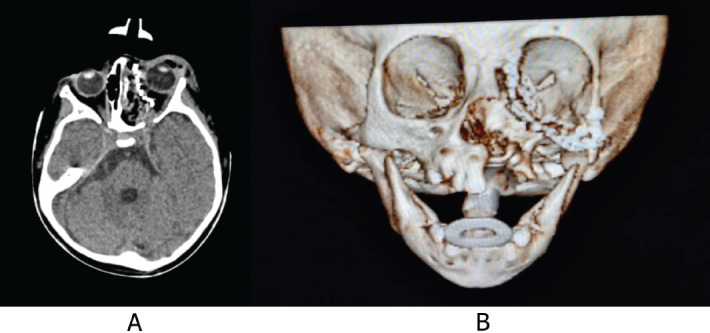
(a): CT scan of the skull in axial view. Post-surgical changes are observed, with no evidence of remaining tumour lesion. (b): Simple skull CT with 3D reconstruction post-surgical control. Post-surgical changes in the bone tissue are observed, with the reconstruction of the left orbital floor with prosthetic mesh properly placed. Source: Medical registry.
